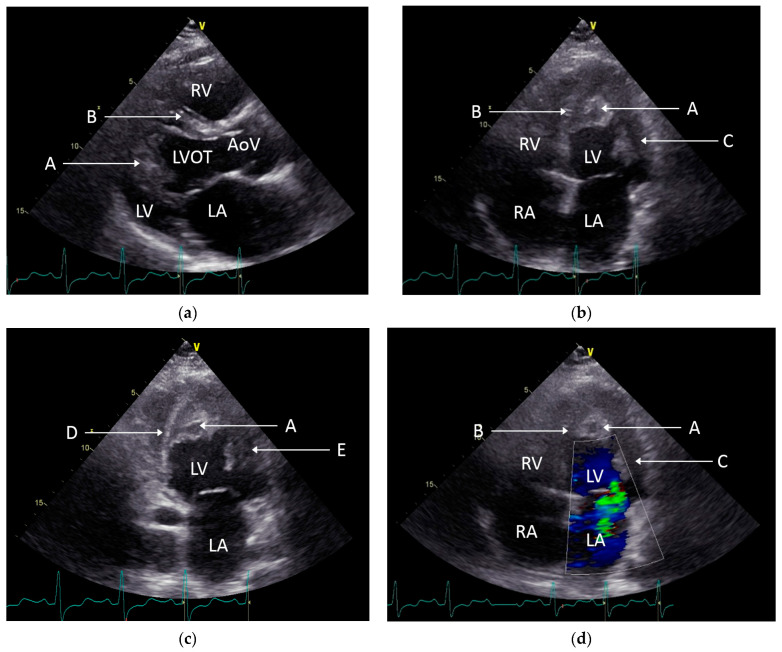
Bidimensional TTE images. (a) The parasternal long axis revealing the presence and extension of the intraventricular mass towards the posterior wall and subvalvular apparatus. (b) Apical four-chamber view (A4C) depicting the hyperechogenic structure obliterating the apex and lateral wall. (c) Apical two-chamber view (A2C) showing the extent of the intraventricular mass to the anterior wall of LV. (d) A4C and color doppler showing moderate-to-severe mitral regurgitation. A—intraventricular mass, AoV—aortic valve, B—interventricular septum, C—lateral wall of the left ventricle, D—inferior wall of the left ventricle, E—anterior wall of the left ventricle, LA—left atrium, LV—left ventricle, LVOT—left ventricular outflow tract, RA—right atrium, and RV—right ventricle.
